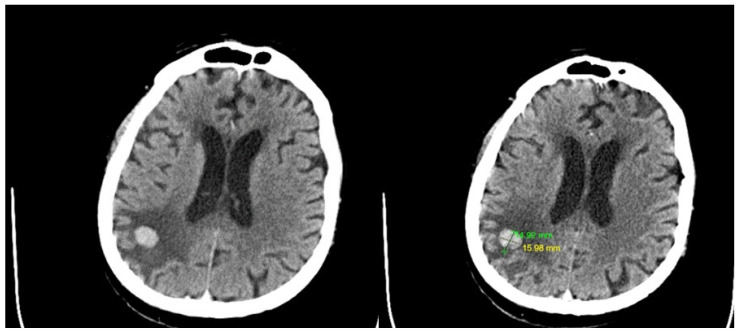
Preoperative contrast-enhanced CT scan revealed a dense, iodophilic nodule measuring approximately 16/14 mm in the right parietal subcortical area associated with digitiform edema in the adjacent white matter. No other pathological contrast enhancements were observed within the brain parenchyma. Leukoaraiosis was present, but there were no intra- or pericerebral hemorrhagic accumulations.
